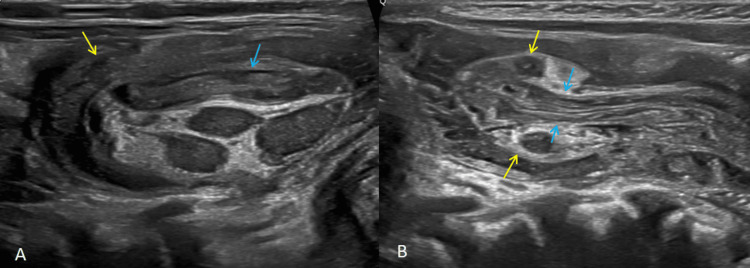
Ultrasound of the abdomen demonstrating ileocolic intussusception, showing telescoping of bowel loops with the characteristic “target” appearance. The yellow arrow highlights intussuscipiens, and the blue arrow highlights intussusceptum.
